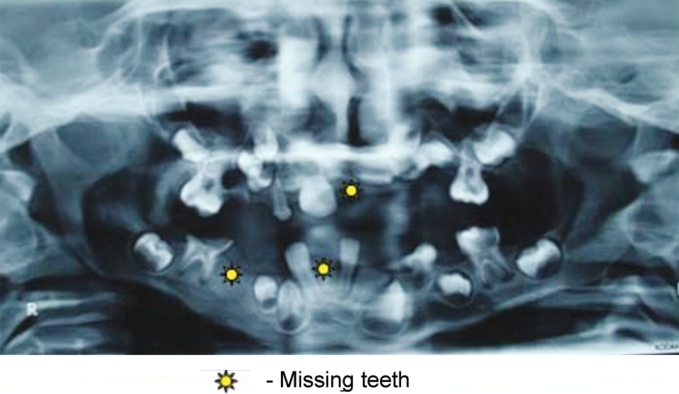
Orthopantomograph showing hypodontia
